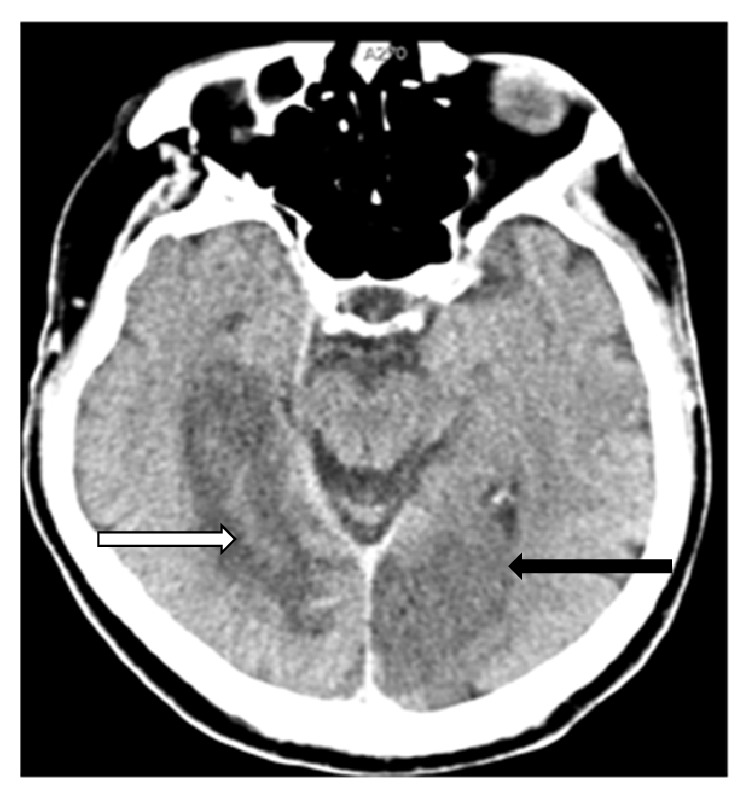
An axial CT image of the brain shows subacute cerebral infarction in the right posterior cerebral artery territory (*white arrow*) and new acute cerebral infarction in the left posterior cerebral artery territory (*black arrow*).
